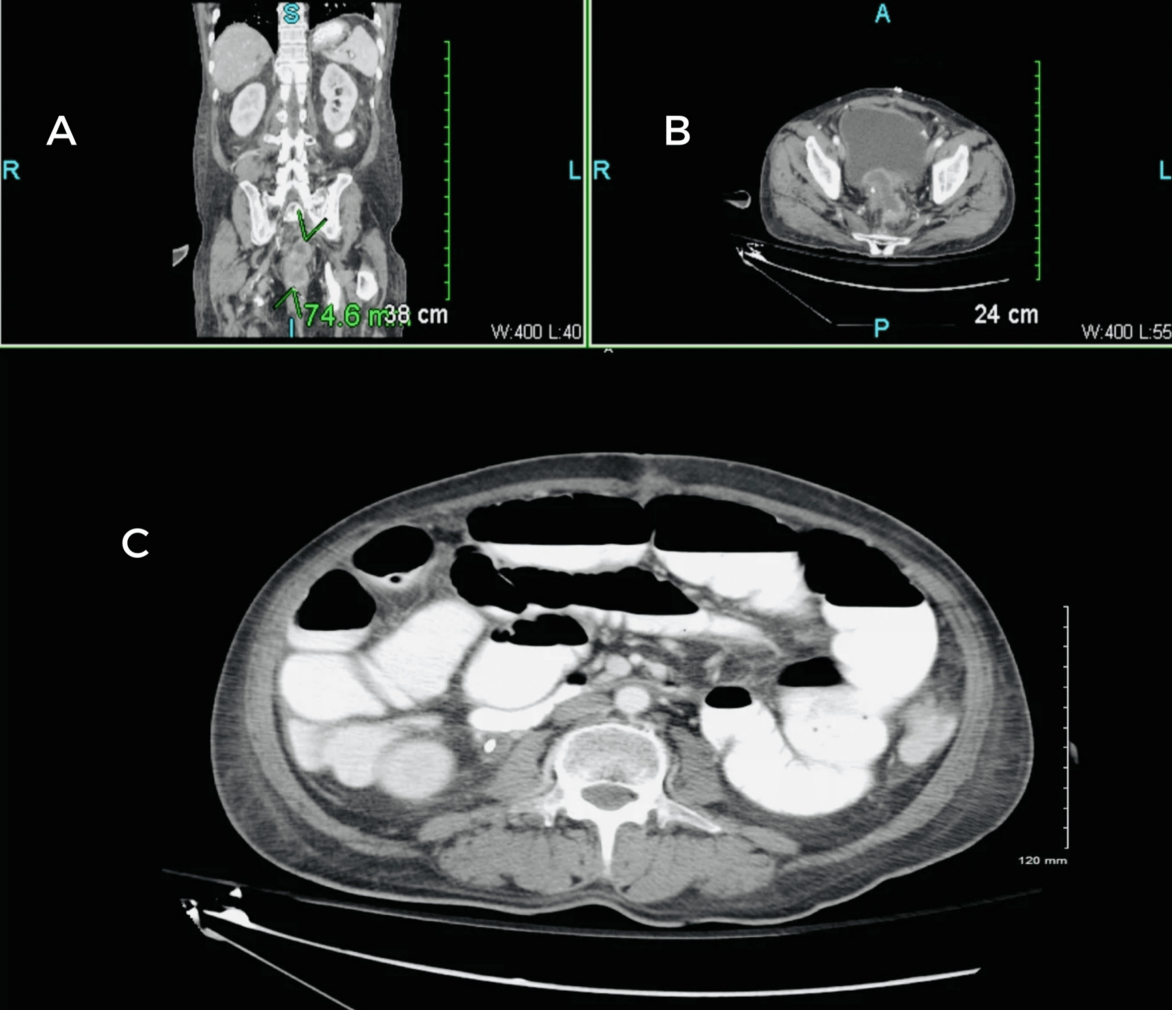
Case two: abdomen CT findings include (A) many metastatic liver lesions of different persuasions; (B) a 7.0 x 4.6 x 7.57 irregular necrotic of the rectosigmoid with necrotic ela area extending bladder wall; (C) moderate distribution of gas and air-fluid levels in the small bowel loops within the abdomen consistent with obstruction.
